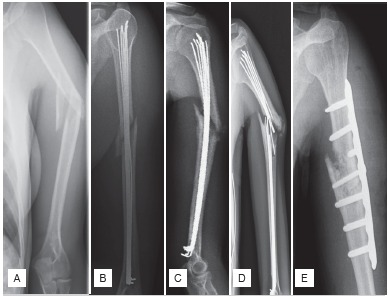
(A) Anteroposterior radiograph of a 37 year old female with an AO type 12-C3 mid-diaphyseal fracture of the humerus. (B) A radiograph obtained two days after surgery demonstrates that the medullar cavity is filled incompletely with implants and a slight distraction of the fragments; (C) Follow-up at 6 months after surgery shows no signs of bony consolidation with slight dislocation; (D) Follow-up radiograph at 18 months shows hypertrophic non-union with further dislocation and hardware failure. Non-union was treated surgically with removal of the elastic nails, resection of the pseudoarthrosis and interlocking plate fixation with cancellous bone grafting from the iliac crest; (E) At 21 months (3 months after revision surgery), union was finally obtained.
